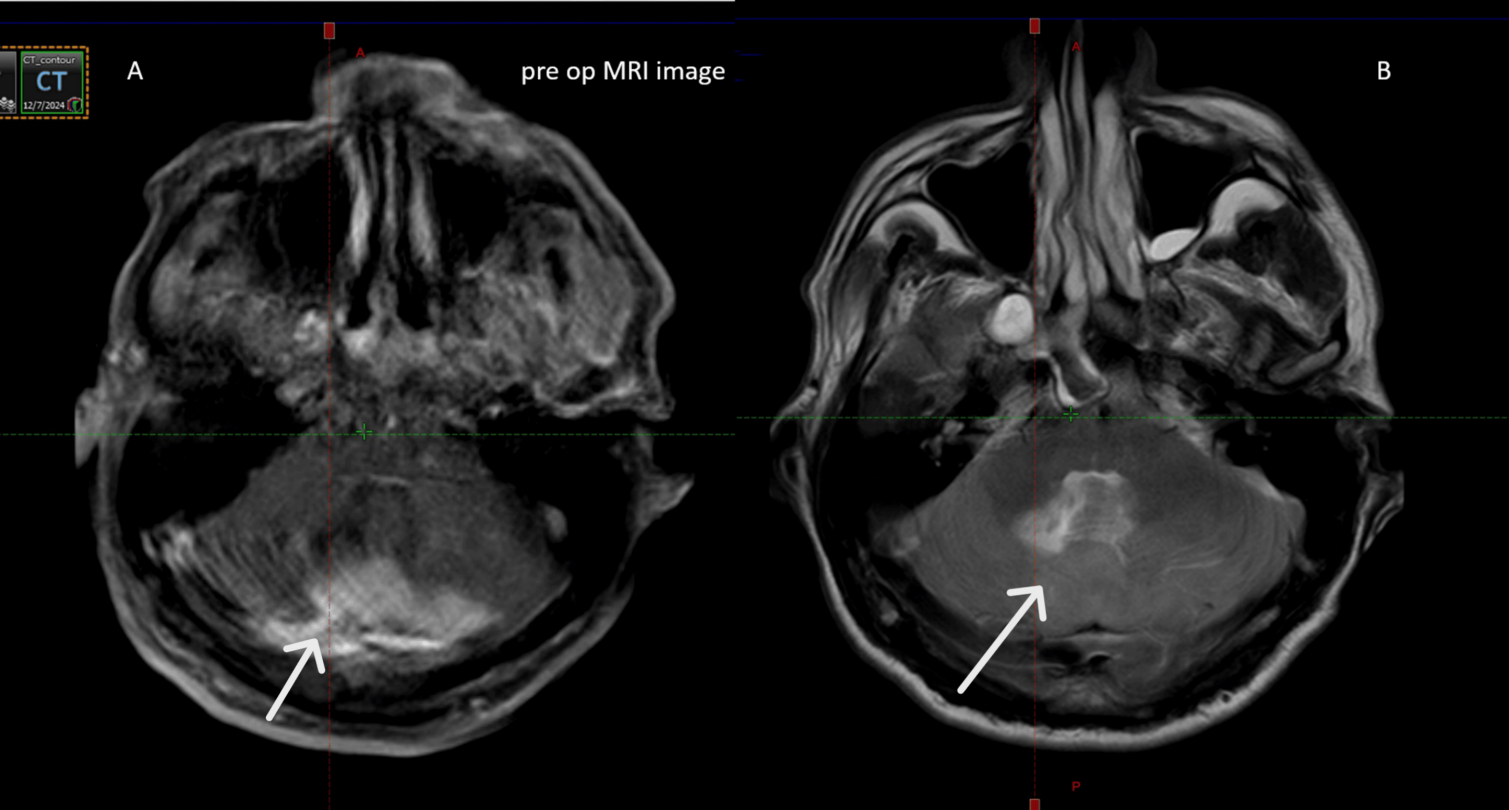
(A, B) Preoperative MRI (axial cuts) showing a hyperdense lesion suggestive of an atypical meningioma (arrows).
